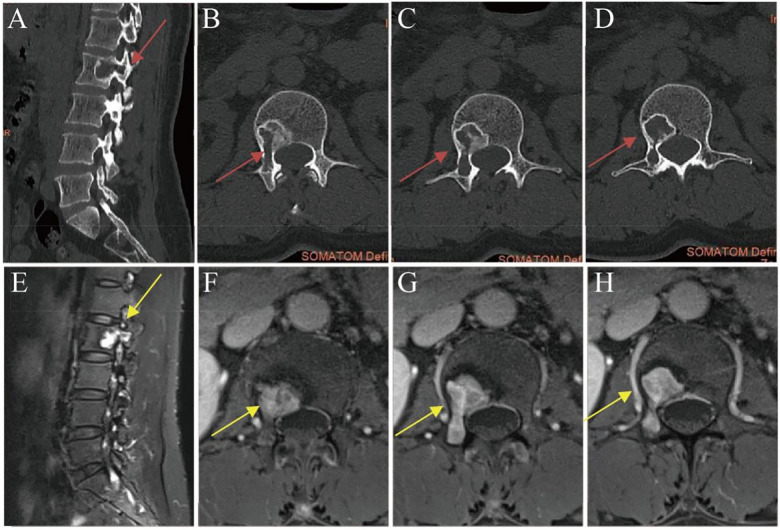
(A–D) Represent preoperative CT scans: irregular osseous destruction area is observed in the L2 vertebral body and its right appendage (red arrows). Images (E–H) show preoperative MRI scans: Irregular abnormal signal changes are visible in the L2 vertebral body and its right appendage, with T2 fat-suppressed images demonstrating uneven high signal intensity (yellow arrows).
